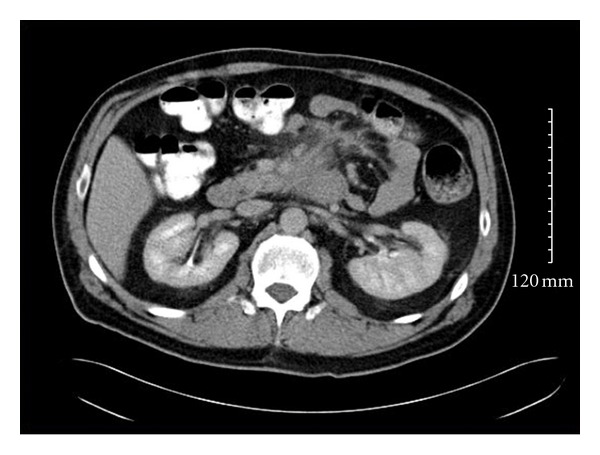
CT abdomen after 1 cycle of chemotherapy showing a decrease in size (2.9 cm × 3.5 cm × 4.4 cm tissue mass) in the distal duodenal mass.
